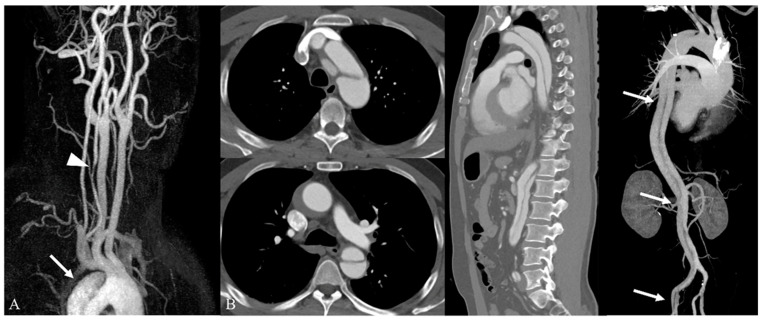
Incidentally detected aortic dissection (Stanford type A) during work-up for dizziness and left arm weakness. (A) In contrast-enhanced neck MR angiography (3D MIP image), there is a double-lumen lesion with intimal flap (arrow), suggesting true and false lumens in the mid to distal aortic arch involving the proximal descending aorta within the scan range. Coexisting focal stenosis is also noted in the left vertebral artery, V2 segment (arrowhead); (B) in contrast-enhanced aorta CT, the Stanford type A aortic dissection involves the whole aorta and extends to the left common and left external iliac arteries (arrows).
